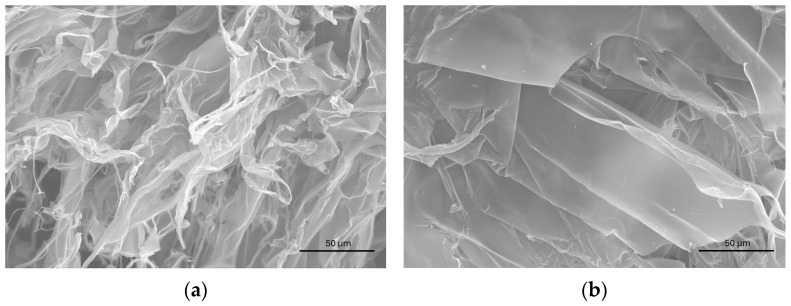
SEM images from CT-DNA. Filament type (a) and plate type (b).
